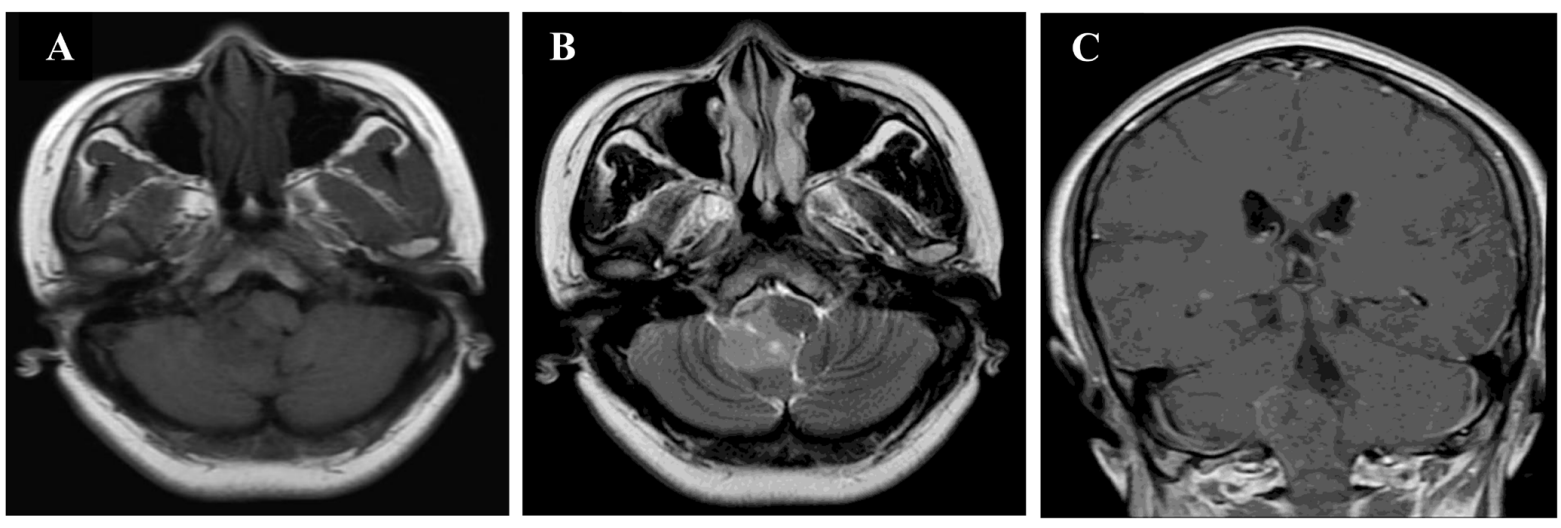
MRI findings in patient 2 A., B., C. Brain MRI (axial T1WI and T2WI, coronal T1WI with contrast enhancement). These images showed an irregular lesion filling up the fourth ventricle, and extending down along the cerebellar medullary fissure. The lesion was isointensity on T1 and T2WI with microcysts. Contrast enhancement was slightly observed in the lesion.
